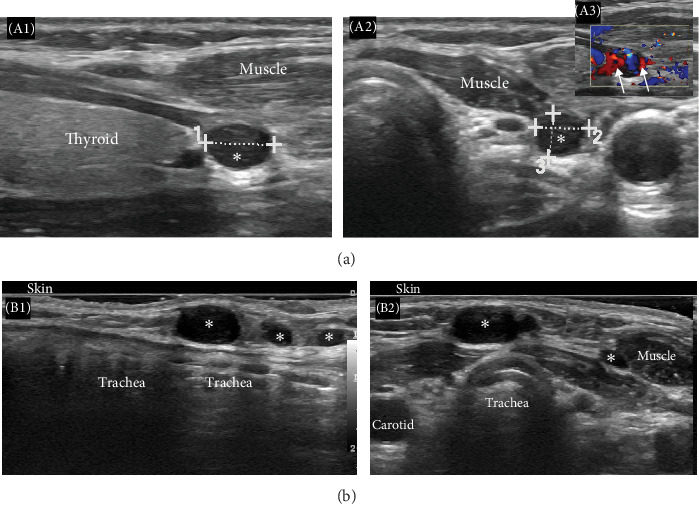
(A) Recurrent hyperparathyroidism 2 years after parathyroidectomy. Longitudinal (A1, B1) and transverse (A2, A3, and B2) grayscale ultrasound images of the left thyroid area. There is a 6.5 mm × 5 mm × 4.2 mm oval well circumscribed hypoechoic nodule (*⁣*^*∗*^ in subparts (A1, A2)) adjacent to the lower pole of the left thyroid lobe, at the site of previous parathyroidectomy. It shows increased vascularity on Doppler (arrows in subpart (A3)). Ultrasound characteristics are identical to the resected parathyroid adenoma and represent recurrent disease. (B) Recurrent hyperparathyroidism 2 years after parathyroidectomy. Longitudinal (B1) and transverse (B2) gray scale ultrasound images of the pretracheal area. There are multiple oval well circumscribed hypoechoic nodules (*⁣*^*∗*^ in subparts (B1, B2)) in the subcutaneous and muscular planes, the largest measures 12 mm. Ultrasound characteristics are identical to the resected parathyroid adenoma and represent parathyromatosis nodules.
